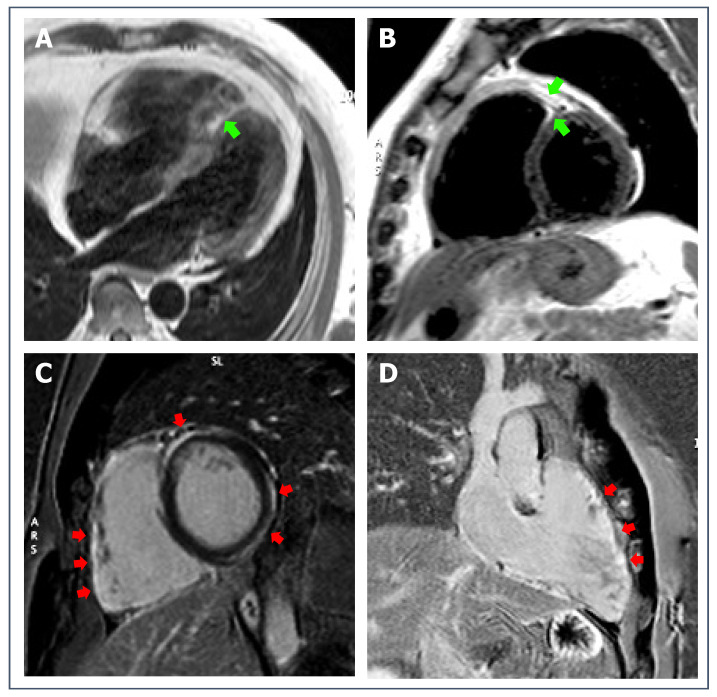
43-year-old patient with biventricular ACM. T1-weighted sequences (A), 4-chambers view, and (B), short-axis view) show mild signs of fatty infiltration (green arrows). Post-contrast sequences (C), short-axis view, and (D), right heart 2-chambers view) reveal non-ischemic LGE involving both RV and LV walls (red arrows).
